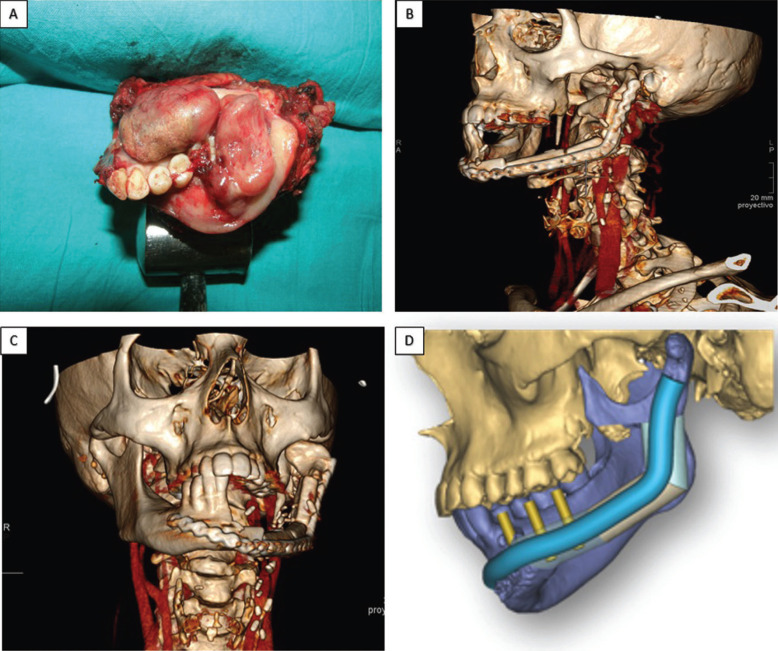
A. Resection piece after excision of the lesion. B,C. CT images captured after reconstruction with microvascularized fibula free flap. D. Planning the surgical reconstruction.
